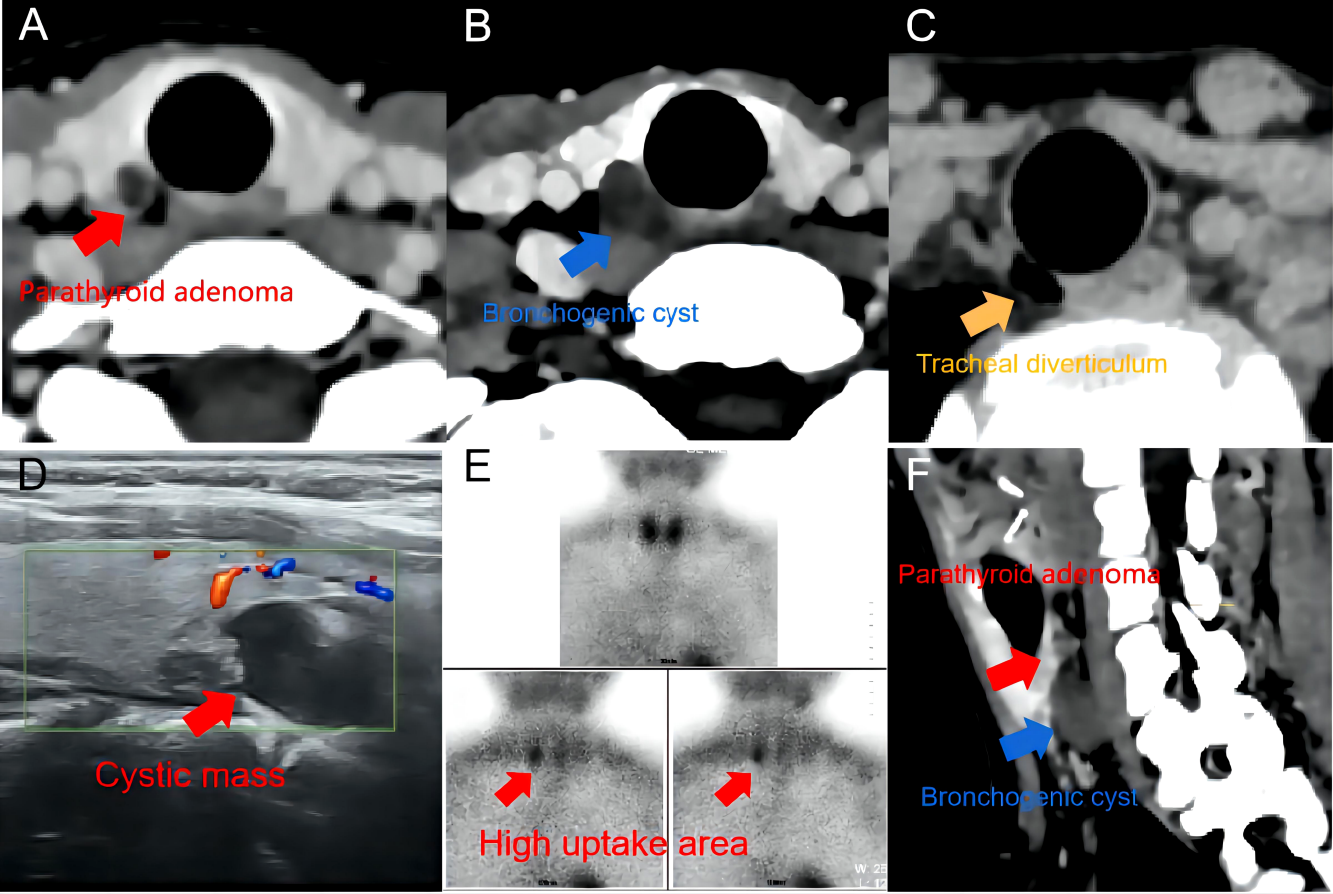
Imaging examination. (A–C) adenoma, cervical bronchogenic cyst, and tracheal diverticulum in CT. (D) a hypoechoic nodule in ultrasound. (E) 99mTc-sestamibi single-photon emission computed tomography: Delayed uptake (120min) in the lower panel suggests a parathyroid source. (F) CT multiplanar reformation (CT-MPR) of the neck.
